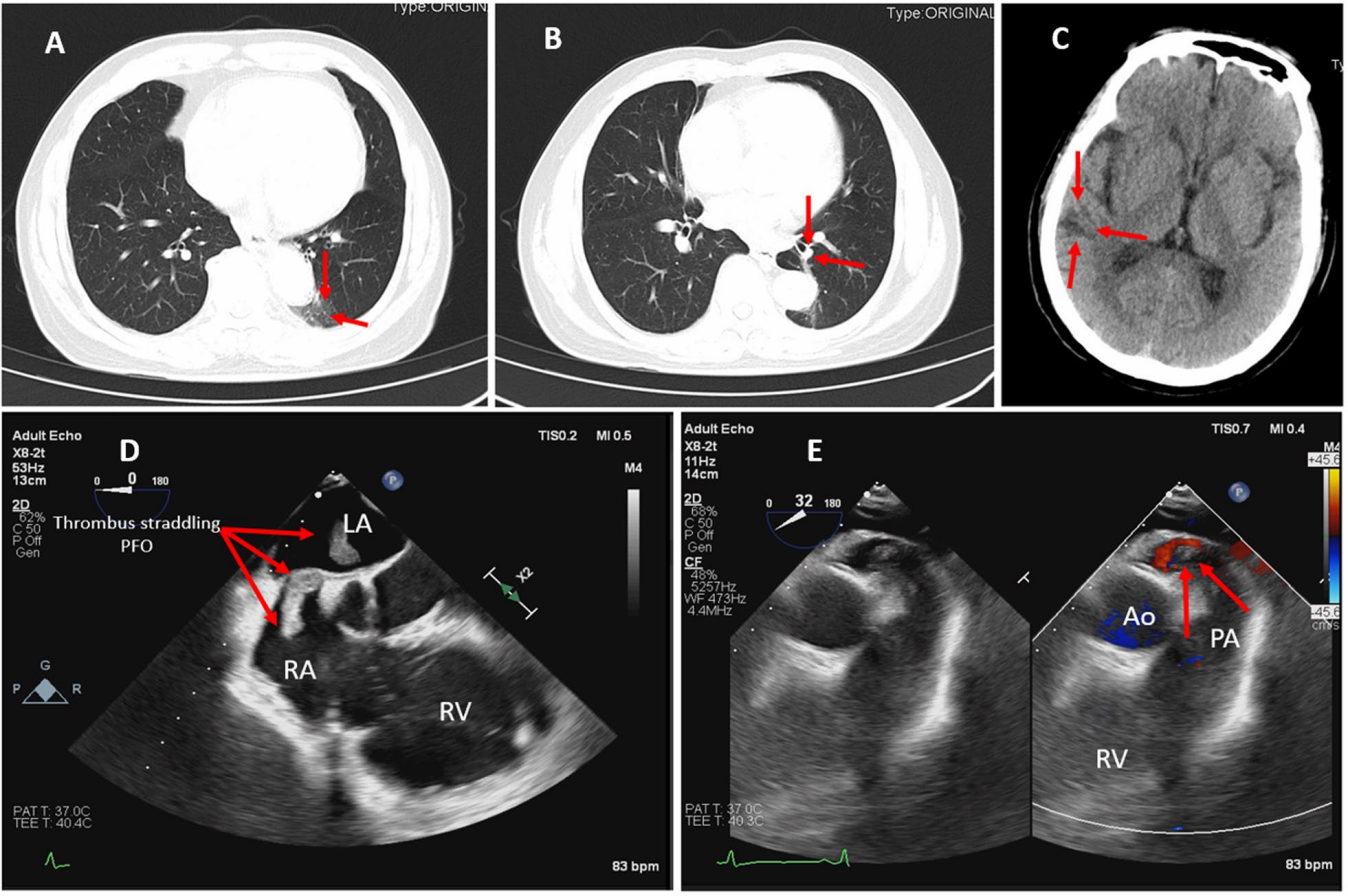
A Nodular shadow in the dorsal segment of the lower lobe of the left lung (red arrow). B Nodular shadow in the basal segment of the lower lobe of the left lung (red arrow). C An irregular patchy area of slightly lower density in the right temporal lobe with unclear and blurred boundaries(red arrow). D Modified four-chamber view: a thrombus traversing the unsealed septal PFO (red arrow), and enlargement of the right ventricle. E. Modified right ventricular outflow tract view: Color Doppler imaging showing a filling defect at the end of the pulmonary artery, suggestive of a thrombus (red arrow). LA: Left Atrium, RA: Right Atrium, RV: Right Ventricle. PFO: Patent Foramen Ovale. PA: Pulmonary Artery, Ao: Aorta
